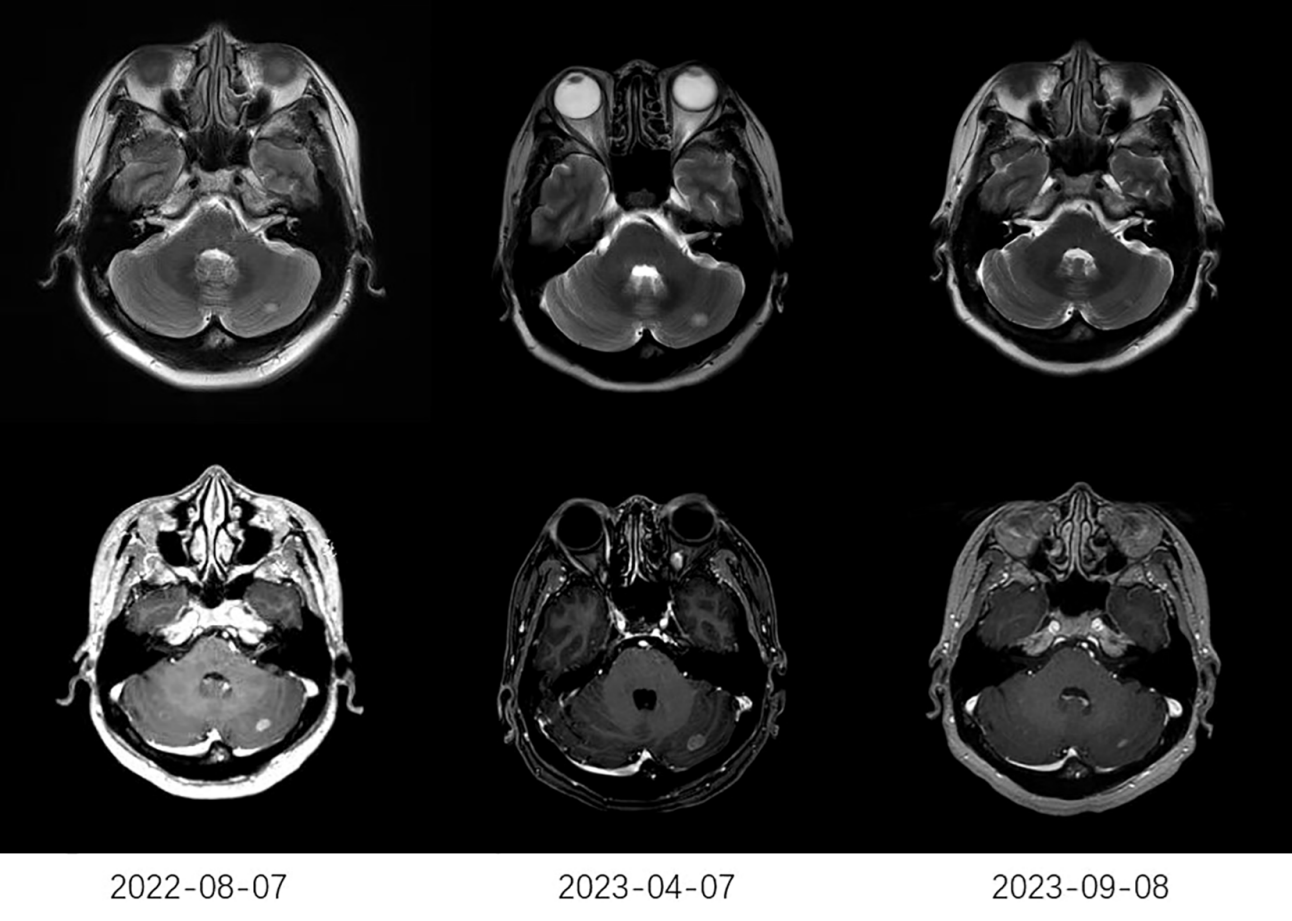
The typical MRI images of one patient. The MRI on April 7, 2023, showed a larger lesion than that on August 7, 2022. After receiving anlotinib (2023-09-08), MRI showed a significant reduction in the lesion compared to that before receiving anlotinib (2023-04-07). MRI, typical magnetic resonance imaging.
